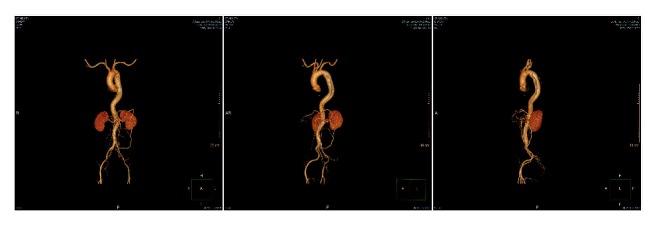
Preoperative CT angiography (CTA) images showing an aortic arch pseudoaneurysm locating between the origin of the left common carotid artery and the left subclavian artery, with a 15-mm tear, 40 mm body and 20 mm sac.
